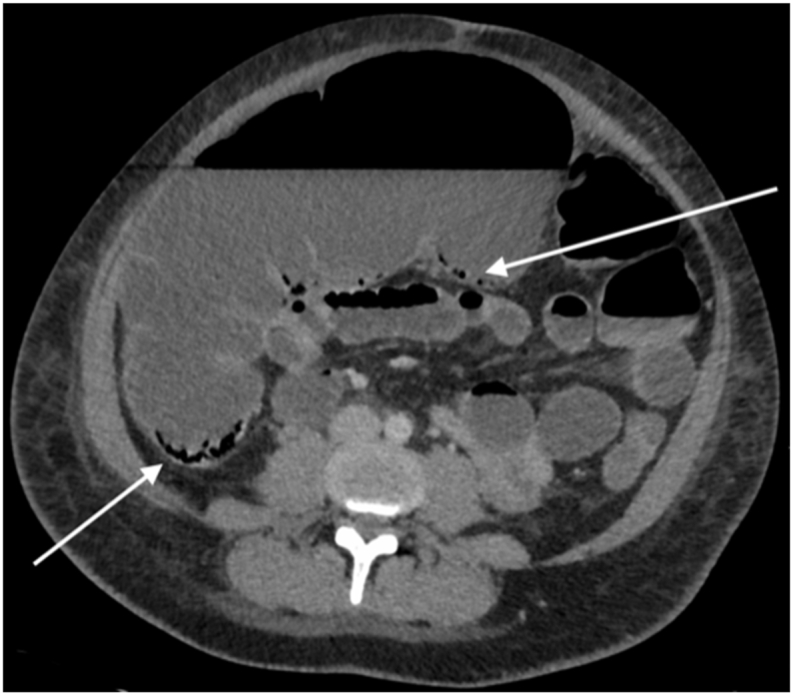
Transversal CT image: Distention of the colon of 10 cm, intestinal pneumatosis is indicated by 2 arrows.
